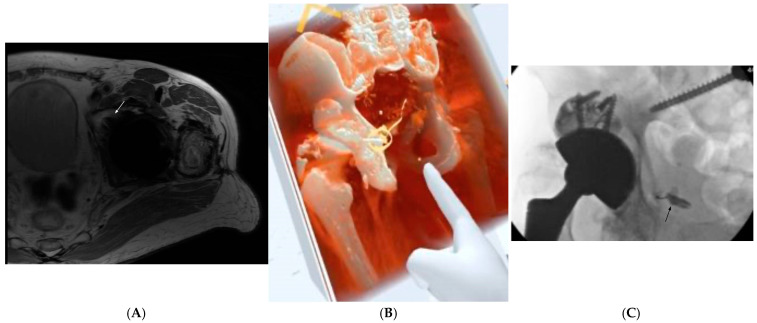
Patient 2, who had persistent pudendal neuralgia following a left total hip replacement and subsequent removal of an acetabular screw causing contact with the pudendal neurovascular bundle. (A) MR of the left hip showed that the acetabular screw (white arrow) was slightly deep and impinging on Alcock’s canal near the ischial spine. (B) Volumetric reconstruction of patient CT imaging following screw removal. Since the primary anatomic landmark for fluoroscopy-guided injection was removed (ischial spine), VR was utilized to obtain measurements and distances to reach Alcock’s canal in order to perform a pudendal block. (C) Fluoroscopy image of successful contrast and medication delivery in the pudendal canal. Arrow indicates block site.
